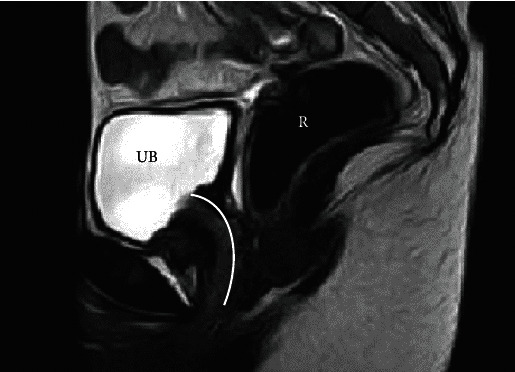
MRI. Sagittal view of pelvis, T2-weighted image, showing urinary bladder (UB), rectum (R), and dilated urethra and bladder neck (line).
